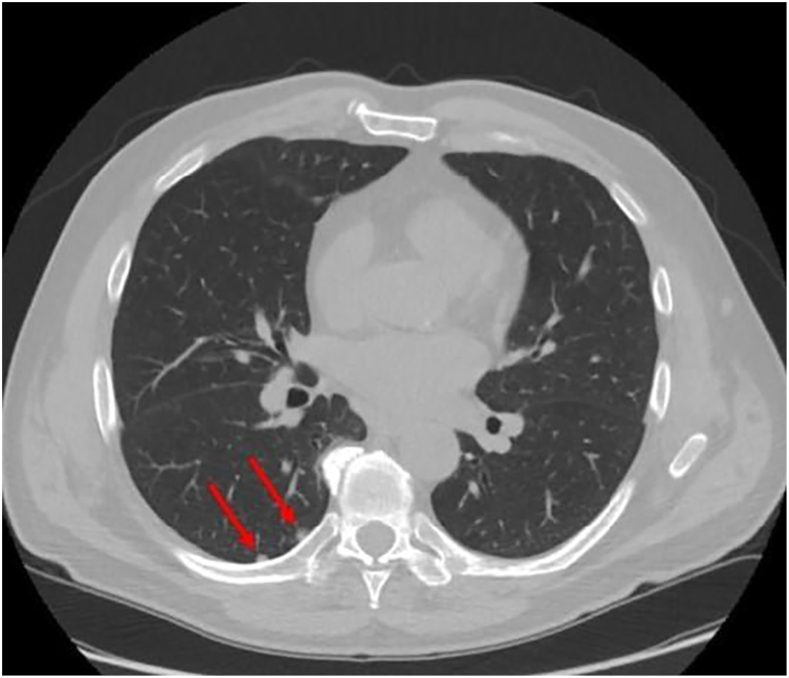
Screening chest computed tomography. Axial image demonstrates 2 subpleural right lower lobe nodules (arrows).
